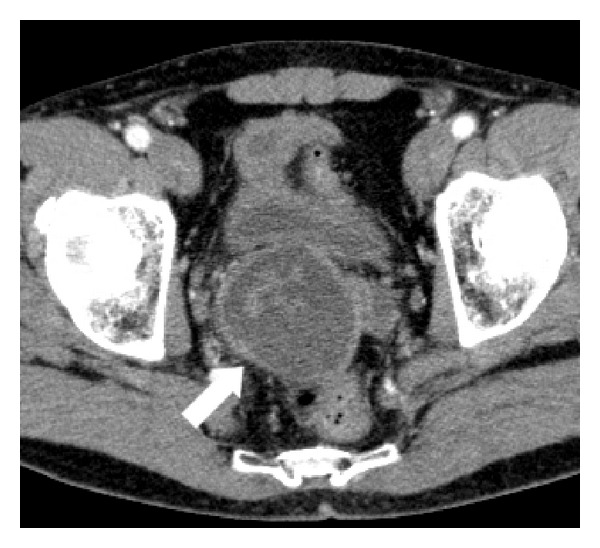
CT. Mixed mass of the right seminal vesicle (arrow).
